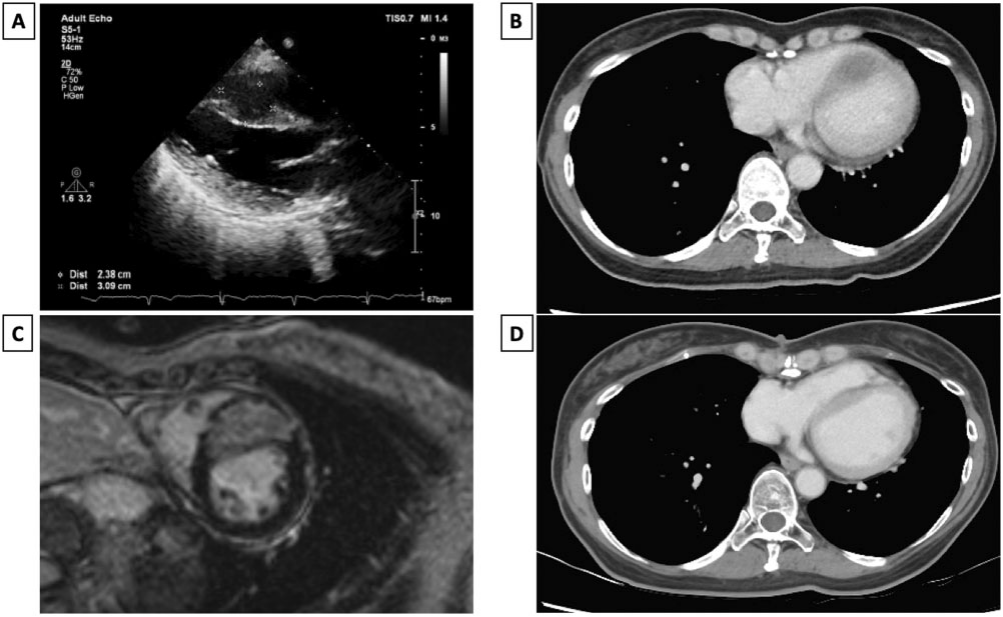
(A) Echocardiography shows a 3.1 × 2.2-cm tumour over the interventricular septum (B) Preoperative axial contrasted chest computed tomography shows a hypodense tumour at the interventricular septum. (C) Cardiac magnetic resonance imaging shows an interventricular mesocardium mass with delayed enhancement and increased T1 value. (D) Postoperative axial contrasted chest computed tomography at the 15-month follow-up.
